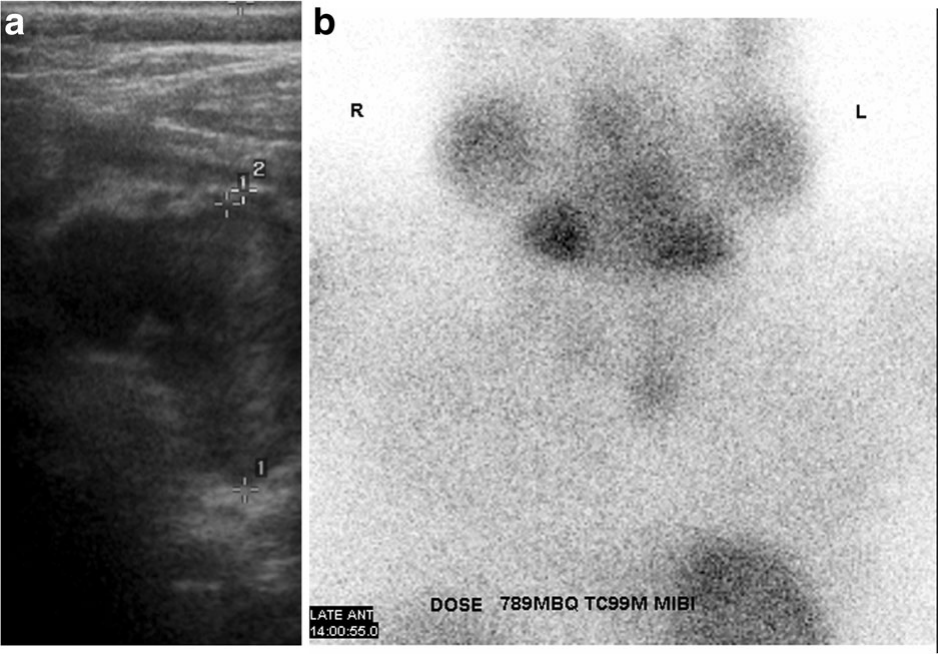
a, b Ultrasound and ^99m^Tc-sestamibi SPECT showed a 2.5-cm left inferior parathyroid adenoma
